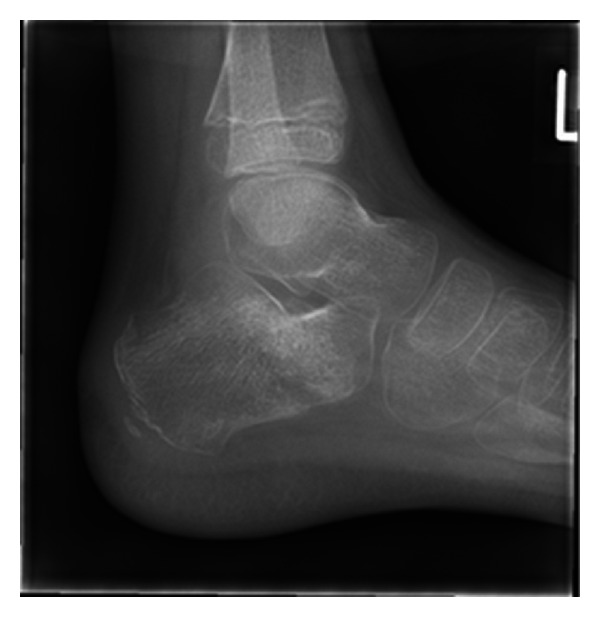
Lateral radiograph at eight-weeks review showing complete bony union.
